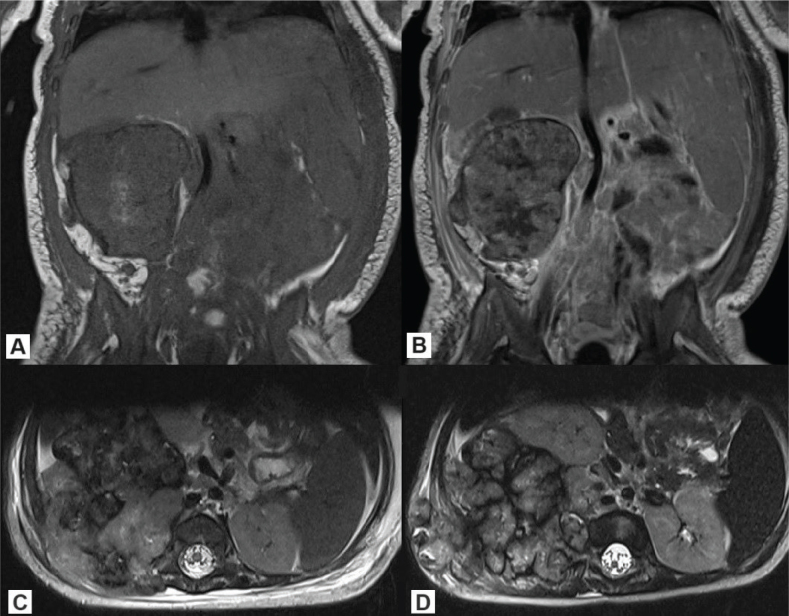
Lumbosacral MRI of case #1: (A) T1 sequence (coronal), (B) T1 contrast enhanced (coronal), (C) T2 sequence (axial) – at the moment of diagnosis; (D) T2 sequence (axial) at disease progression. MRI: Magnetic Resonance Imaging.
